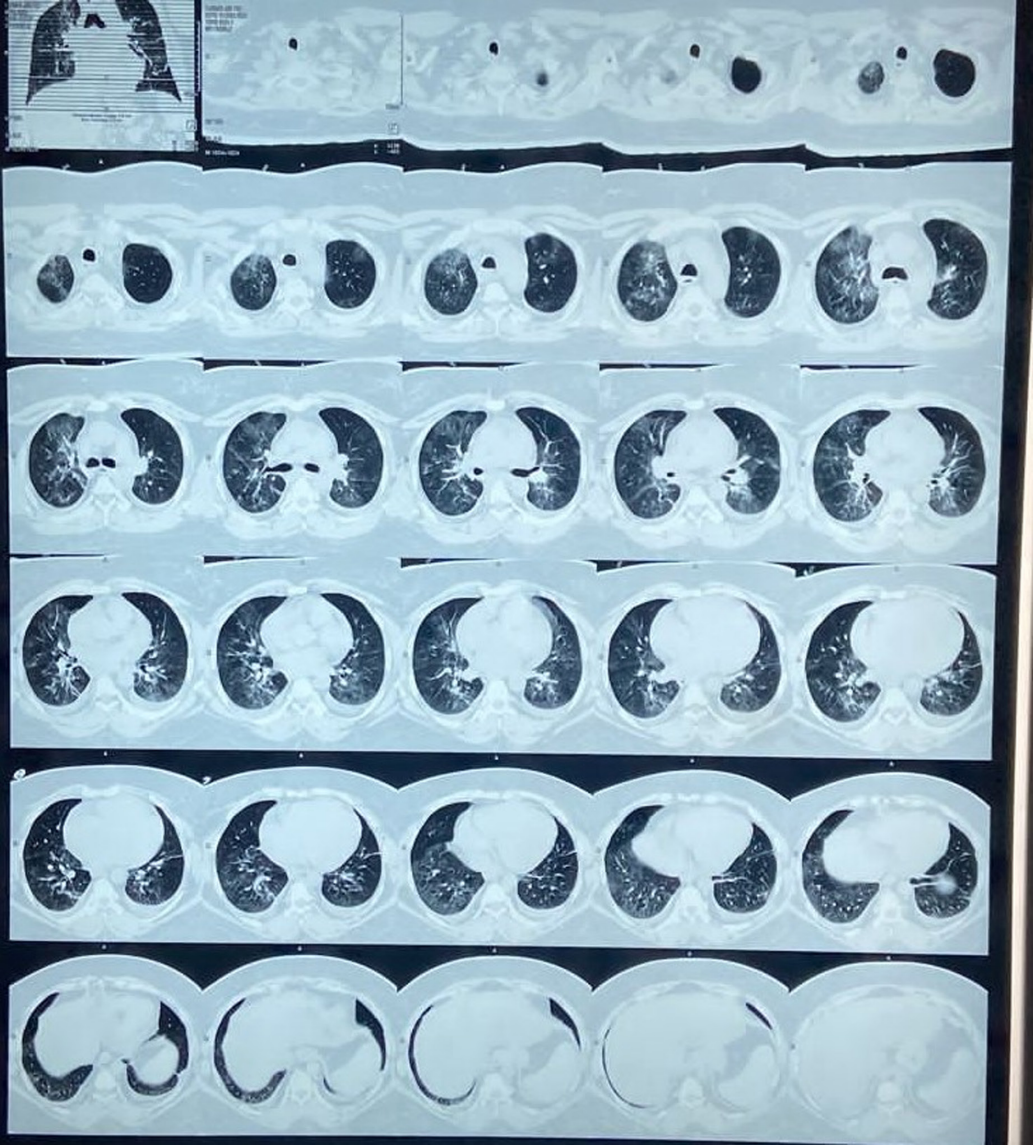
High‐resolution computed tomography (HRCT) of the case
